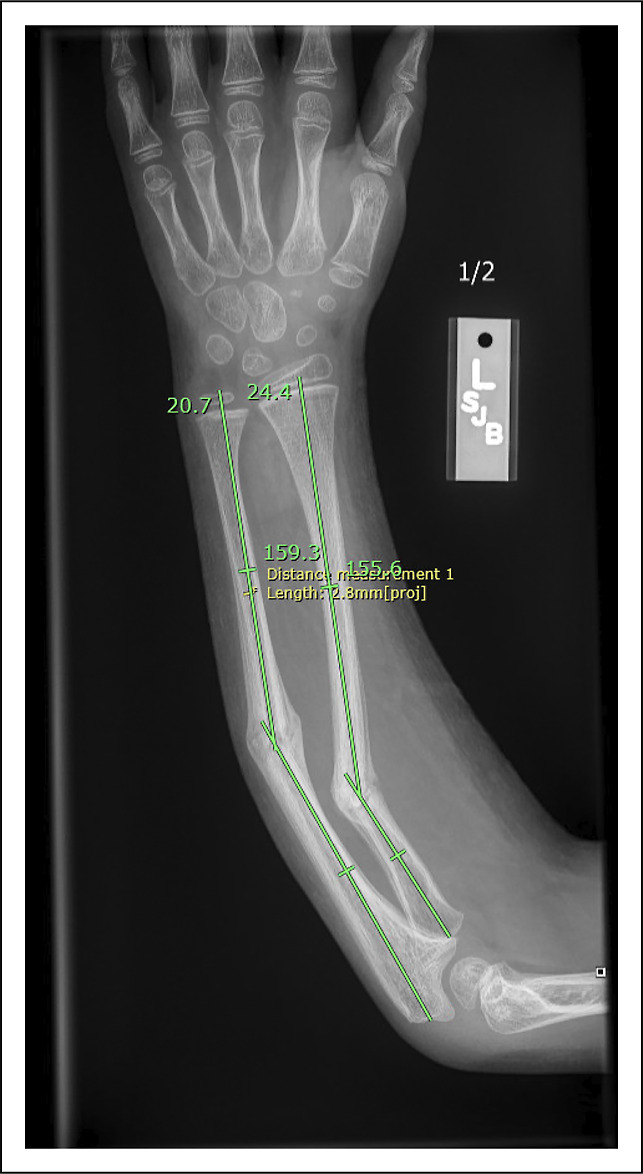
Radiograph showing radial and ulnar diaphyseal malunion after 8 weeks of closed treatment in a long arm fiberglass cast.
